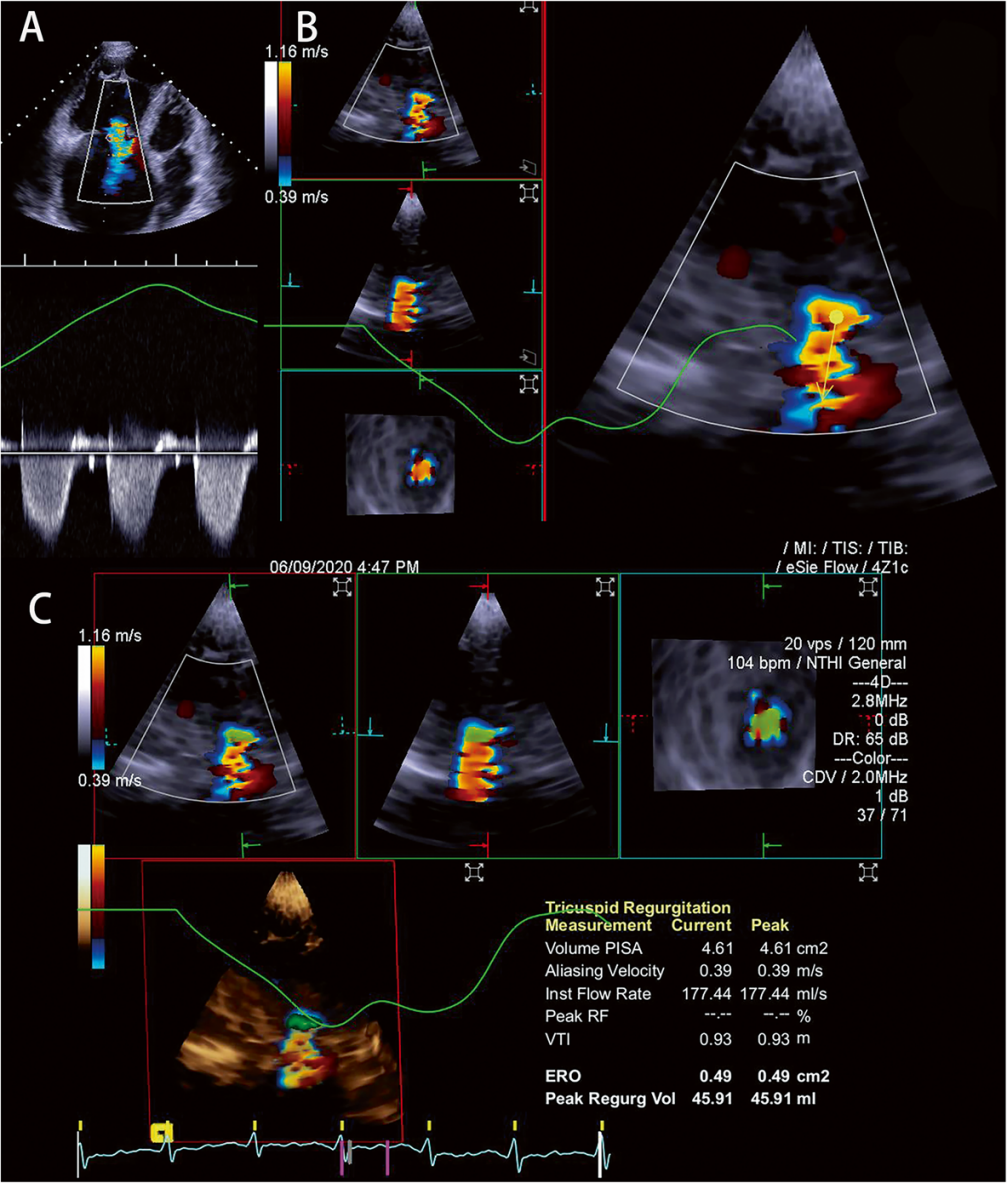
Measurement of 3D PISA EROA. a The TR VTI was measured by continuous-wave Doppler. b The arrow was set in direction of regurgitation. c The full volume ultrasound images were displayed in three orthogonal planes (left, four-chamber view; center, two-chamber view; right, short-axis view). PISA was visualized as green overlay on a 3D color Doppler image. 3D PISA EROA was calculated as (3D PISA x V_aliasing_)/ peak TR velocity
